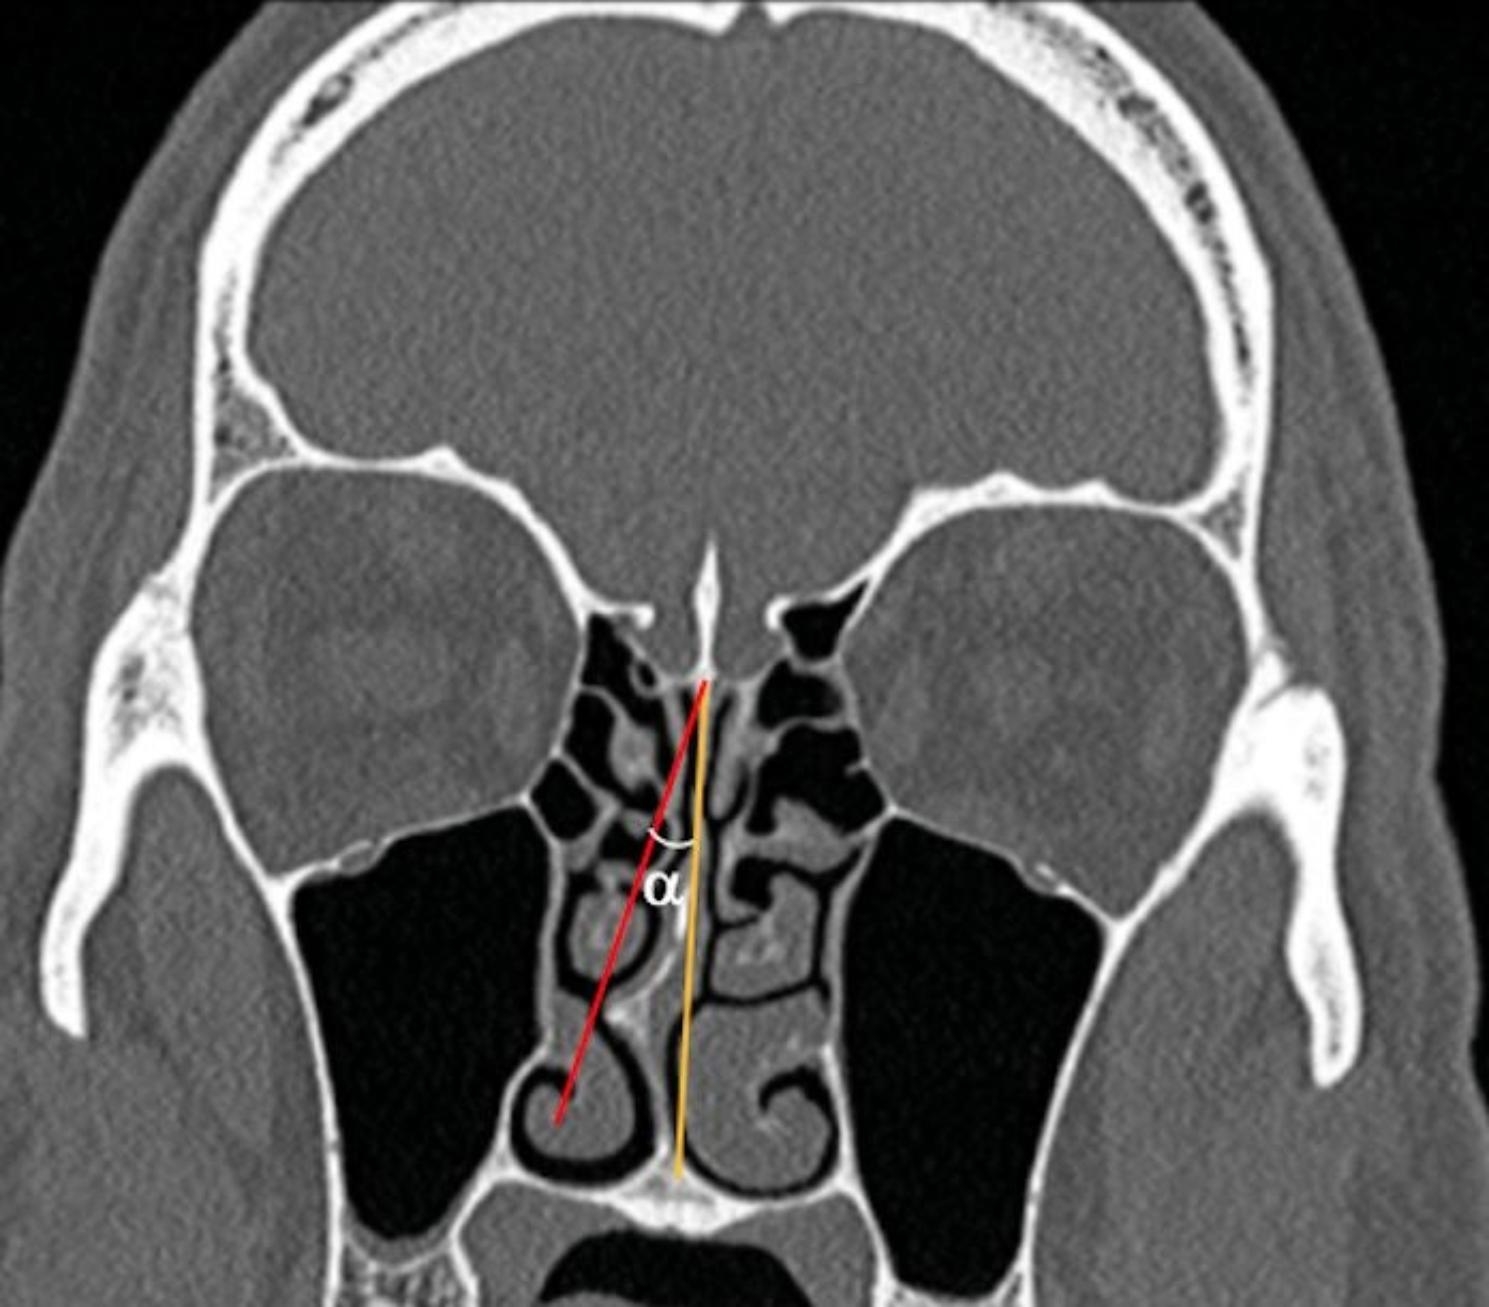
Measurement of NSD Angle on CT
